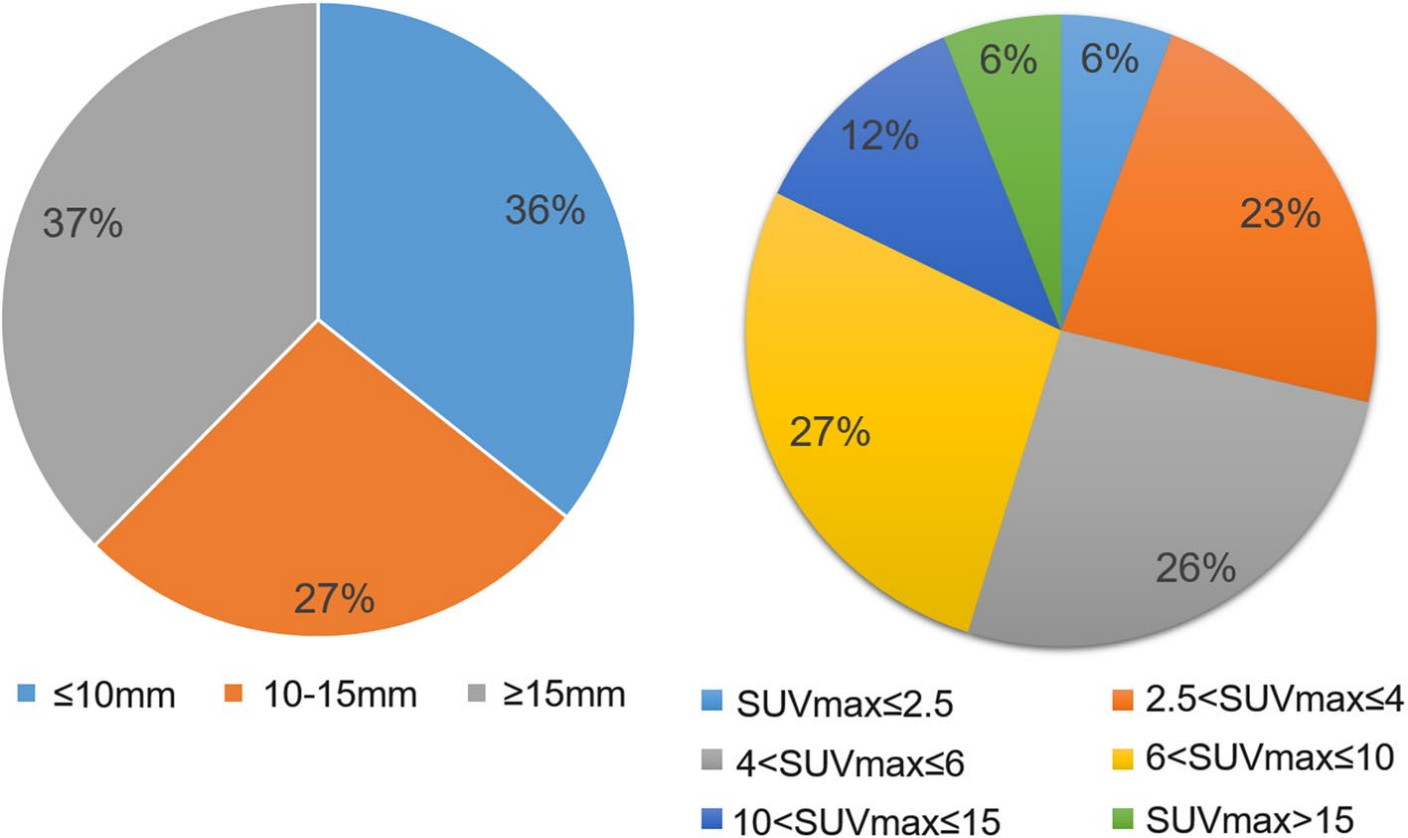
Characteristics of the identified lesions (*n* = 314). Left: Diameters of the lesions measured in computed tomography images. Right: SUVmax of the lesions measured in the reference GS_180 PET images. SUV, standardized uptake value
